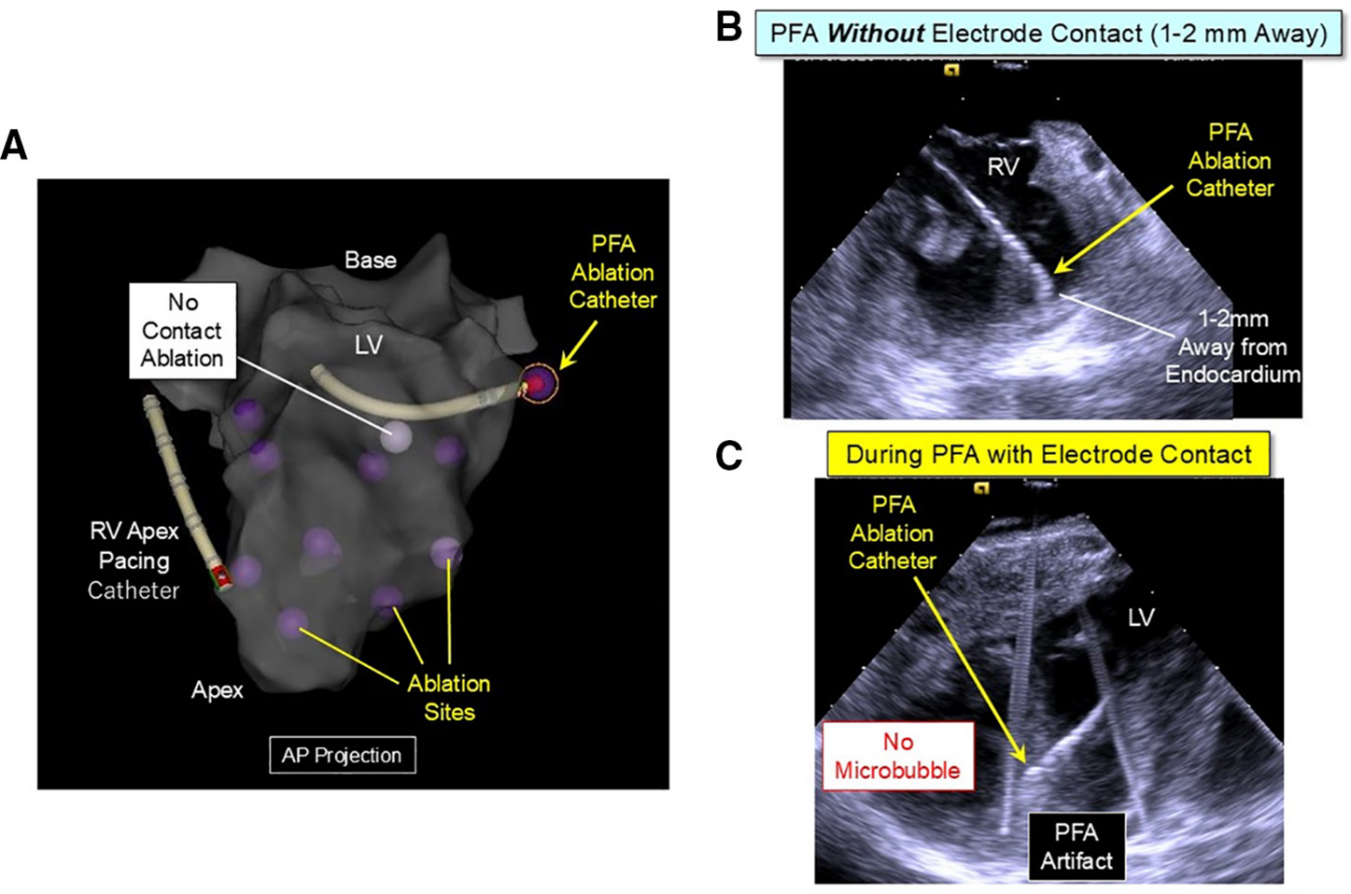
Three-dimensional electro-anatomic map and intracardiac echocardiography of the ventricle demonstrating pulsed field ablation (PFA) sites with and without electrode contact. A, The anatomic shell of the left ventricle (LV) is shown in the anterior-posterior (AP) projection. PFA is performed at separate sites with electrode contact (purple tags) and without contact (white tag) during right ventricle (RV) apex pacing. B, Intracardiac echocardiography showing a PFA catheter. PFA is performed without electrode-myocardium contact, ≈1 to 2 mm away from the endocardium. C, No microbubbles are detected during PFA applications in the LV with electrode contact. White lines indicate the artifact produced by PFA applications.
